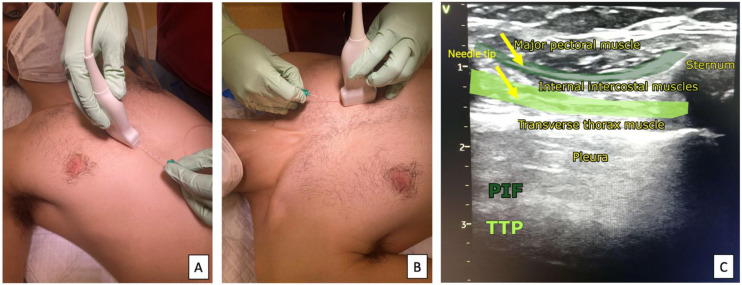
Ultrasound transducer positioning of the TTP (A) and PIF (B) blocks and sonoanatomy of the TTP and PIF blocks (C).
